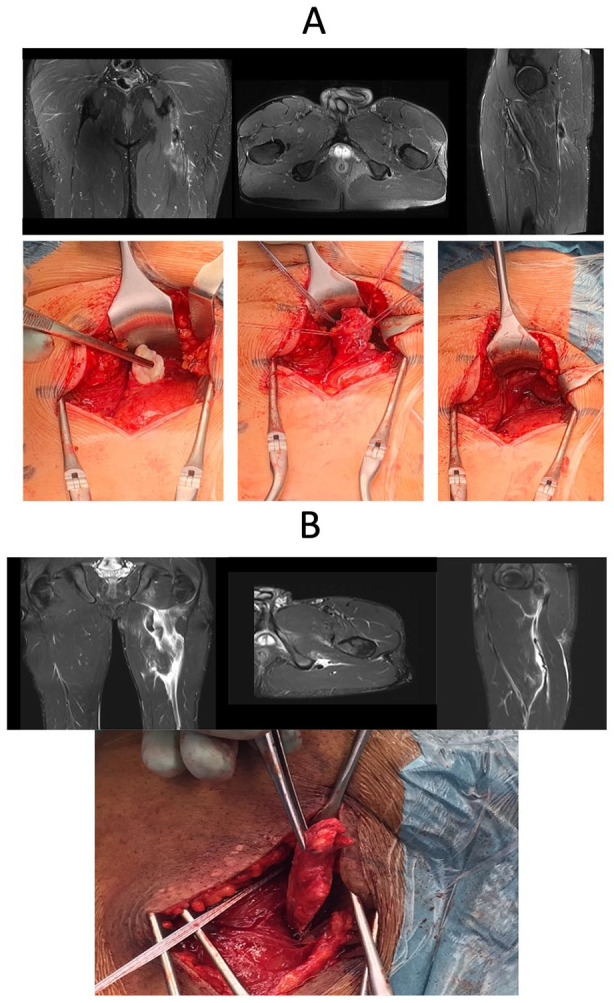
Isolated complete proximal single-tendon rupture: A) BF (MRI and perioperative images), B) SM (MRI and perioperative images).
